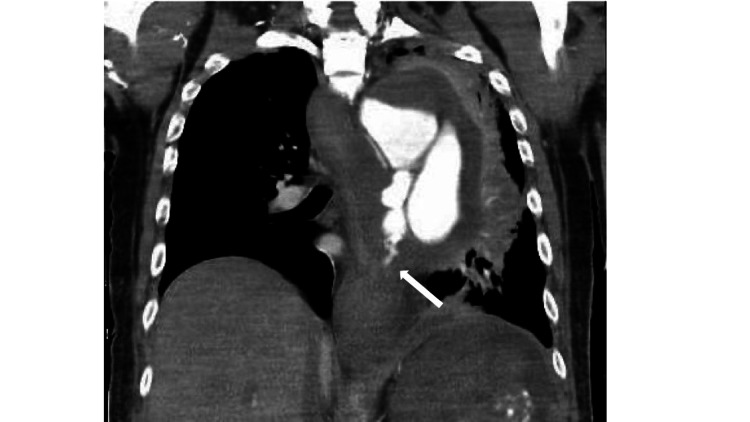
Imaging study of the chest after hospitalization Contrast-enhanced chest CT finding showing approximately 300 mm from the oral cavity to the esophageal perforation (arrow). CT, computed tomography
